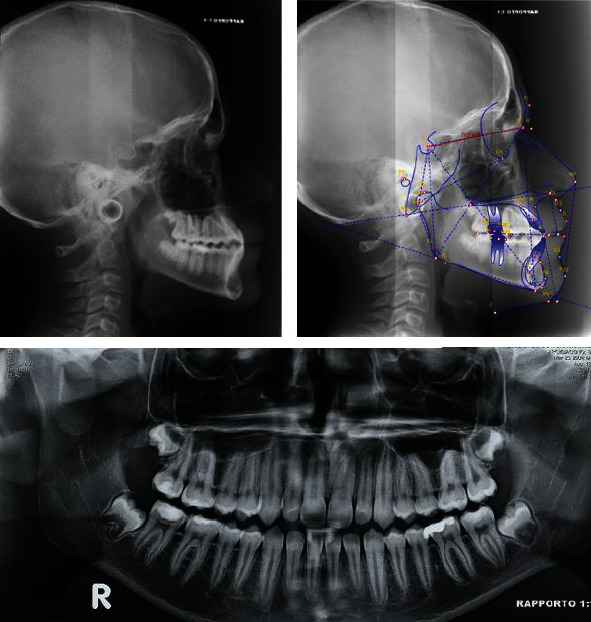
Pretreatment lateral cephalometric radiograph, tracing, and panoramic radiograph.
